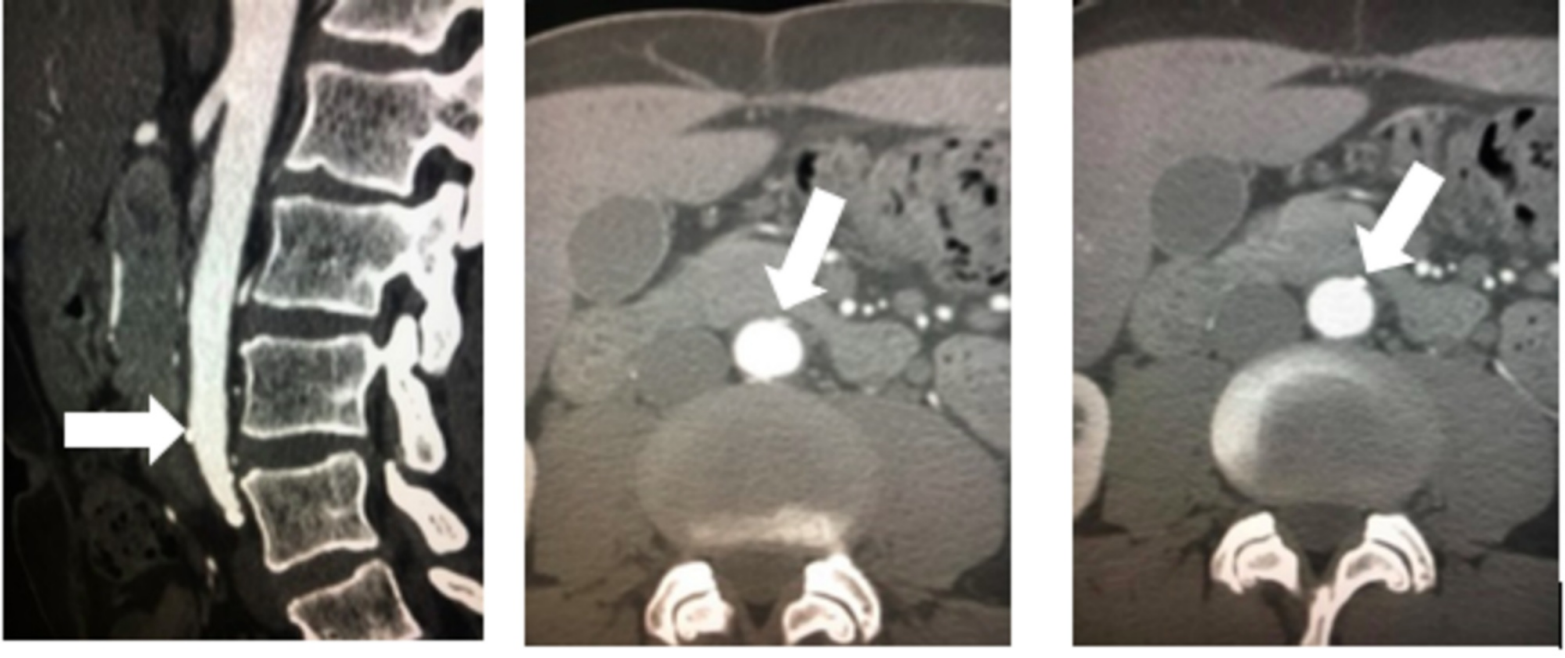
Left to Right: CT angiography showing occlusion of the inferior mesenteric artery, as indicated by the white arrow, at its origin in the sagittal and coronal planes CT: computed tomography
